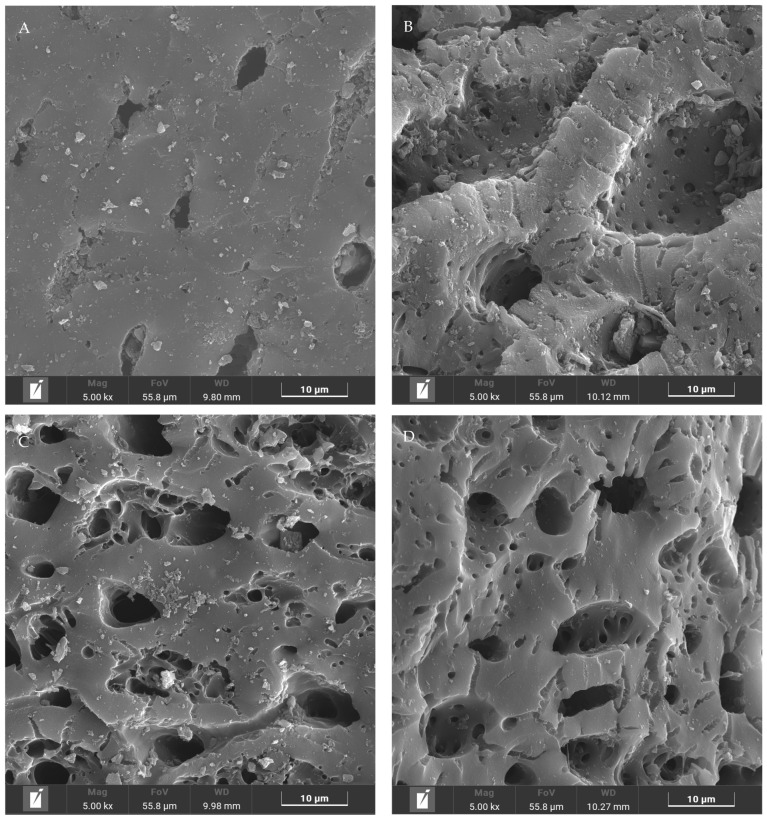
SEM analysis of CAC with different pretreatment. (A) initial state; (B) acid eluting; (C) ultrasonic treatment, and (D) acid eluting under the ultrasonic treatment.
